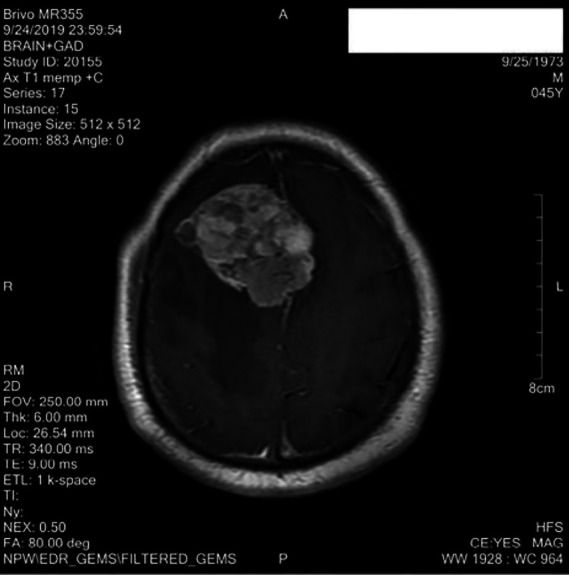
MRI revealing a large metastatic lesion in the right frontal lobe
